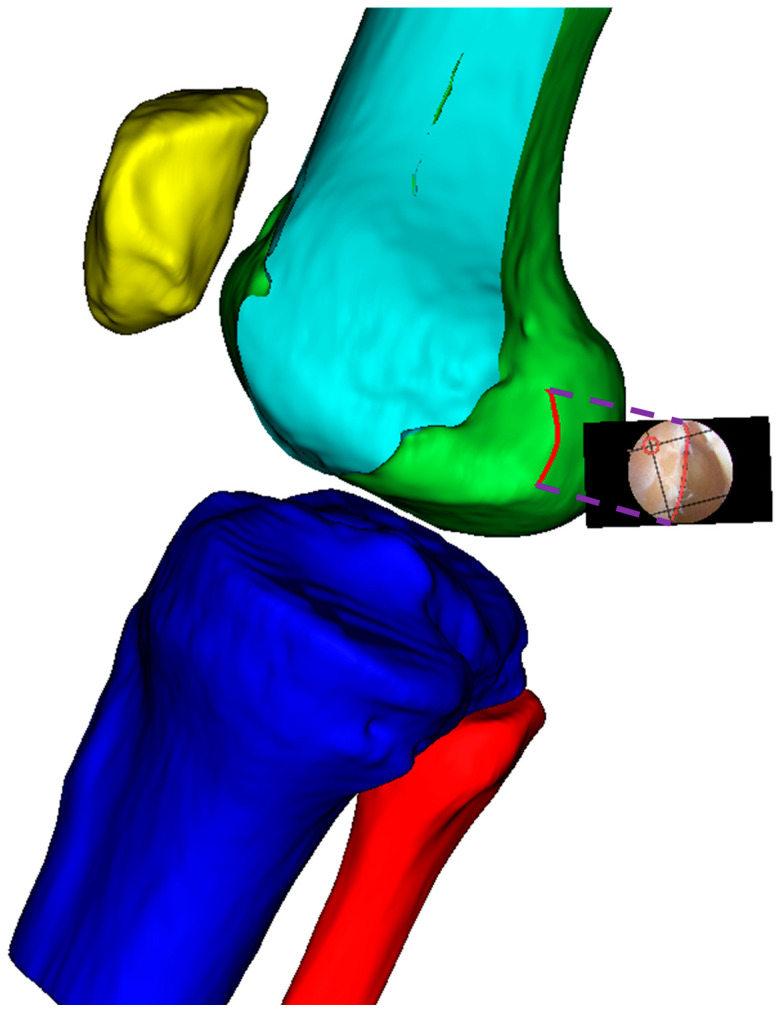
The registration of arthroscopic and preoperative CT images based on reference capsular lines. The registration process employs the SIFT-ICP framework, where SIFT features are first extracted from both imaging modalities for initial alignment, followed by ICP refinement to achieve precise spatial correspondence. The capsular lines serve as anatomical landmarks to guide and validate the registration accuracy.
